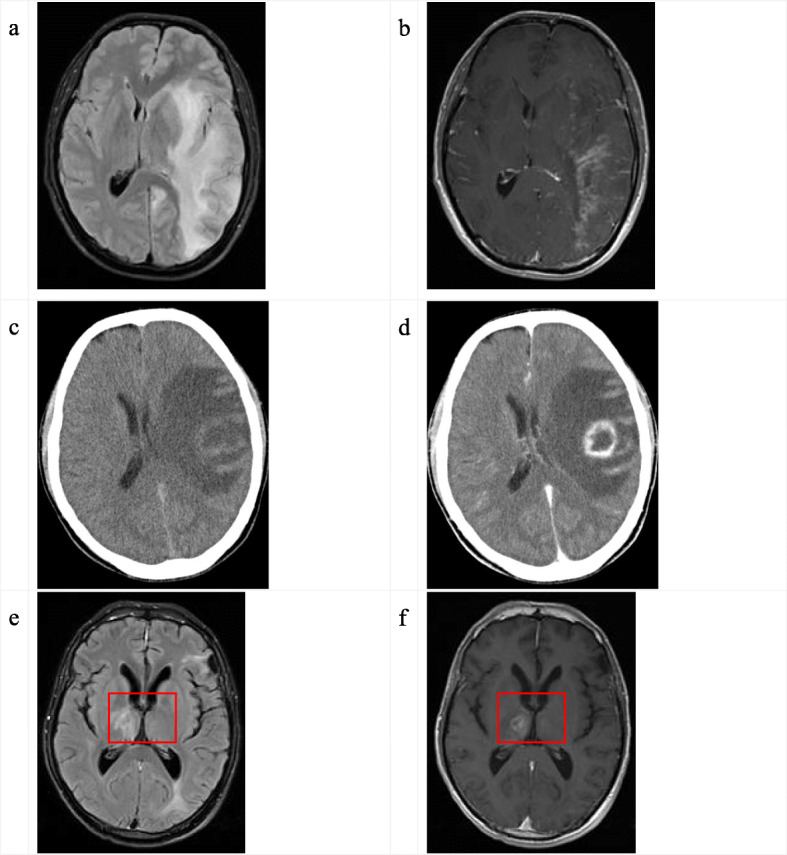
Radiological images of three cases of non-survival. The T2 flair series showed large patchy abnormal signals on the left frontal lobe, temporal lobe, and occipital lobe (a). T1 gadolinium-enhanced scans showed multiple patchy enhancements in the left frontal lobe, temporal lobe, and occipital lobe (b). A CT scan of the head revealed a large patch of low-density shadow on the right temporal lobe (c), and an enhanced CT scan revealed a ring-shaped enhancement on the right temporal lobe (d). The T2 flair series showed slice-like and patch-like abnormal signals at the junction of the right thalamus, left frontal lobe, and left temporal and occipital lobes (e). A T1 gadolinium-enhanced scan showed a ring-shaped enhancement in the right thalamus (f). Note: Lesions on temporal lobe were reported to be related to memory deficits (a-d); lesions on thalamus were reported to be related to disorders of consciousness (e-f).
